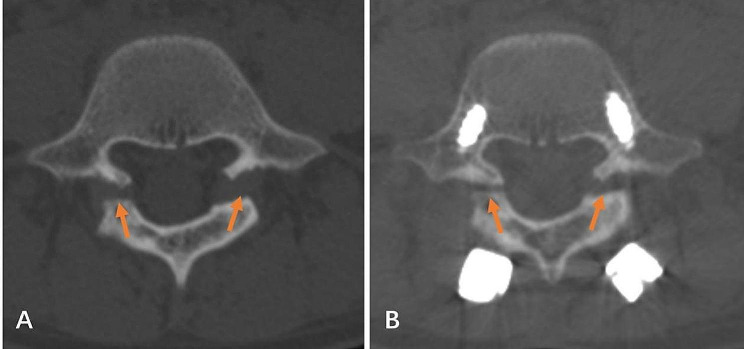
Representative axial CT images of non-fusion of spondylolysis after pars repair surgery. Pre-operative image of a patient with stage III pars defects (A). CT showing bilateral non-fusion at 12 months after pars repair surgery (B)
